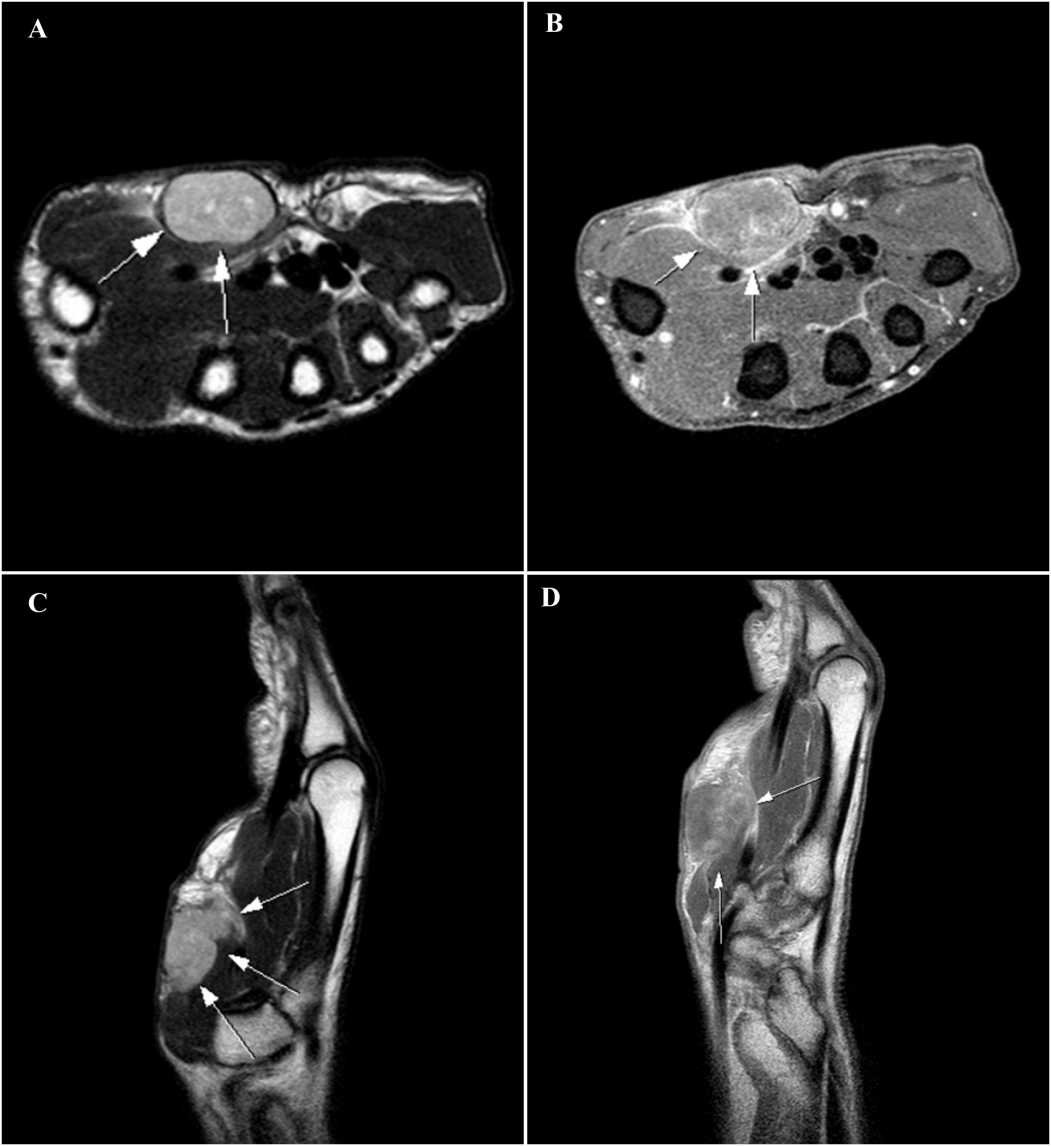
T2-weighted spin-echo sequences without fat saturation in the axial (A) and sagittal (C) planes demonstrate a nodular lesion in the subcutaneous layer of the palmar aspect of the hand. The intermediate signal intensity lesion is oval-shaped, with regular, well-defined margins. On T1-weighted spin-echo sequences with fat saturation in the axial and sagittal planes (B and D), the lesion shows mild contrast enhancement.
